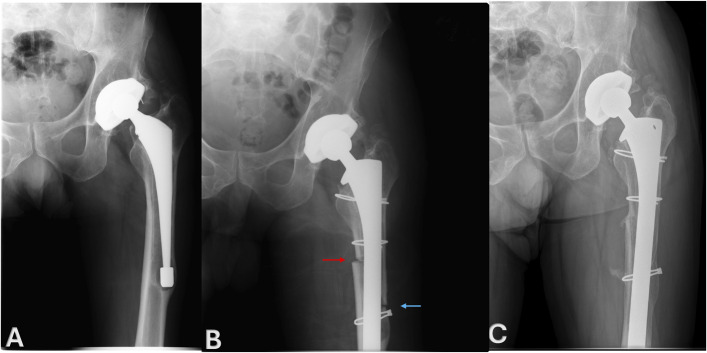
A 54-year-old man who underwent an ETO combined with a medial corticotomy for revision THA due to femoral varus deformity. Fig. 3-A Preoperative AP radiograph showing the varus femoral deformity. Fig. 3-B Immediate postoperative AP radiograph showing the revised femoral stem, the ETO (blue arrow), and the medial corticotomy (red arrow), with correction of the femoral varus deformity. Fig. 3-C AP radiograph 4 years postsurgery, demonstrating complete healing of both the ETO and medial corticotomy and sustained correction of the femoral varus deformity with continued alignment stability. No signs of implant loosening or complications are observed, and the femoral alignment remains well maintained, confirming the long-term success of the combined ETO and medial corticotomy procedure. AP = anteroposterior, ETO = extended trochanteric osteotomy, and THA = total hip arthroplasty.
